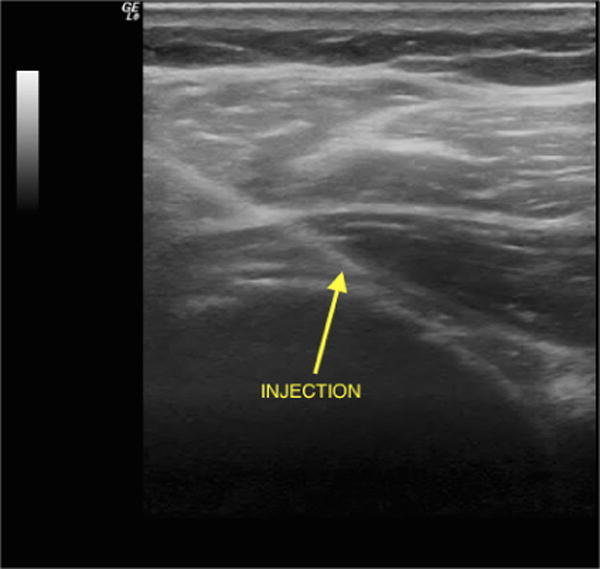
An ultrasound showing the needle approaching the glenohumeral joint
